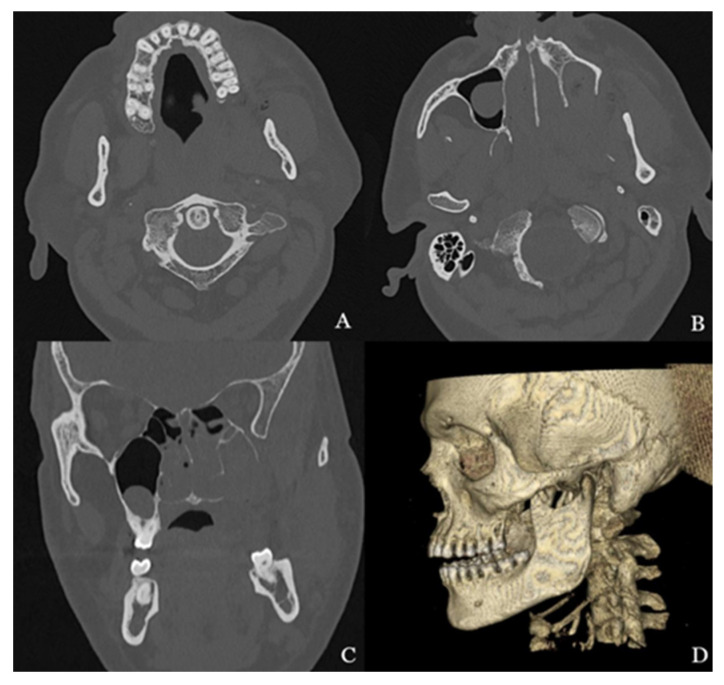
CT scans showing the bone defect in the maxilla after the extraction of tooth 28 ((A,B) axial sections, (C) coronal section), and a 3D reconstruction of the defect (D). The vascular abnormalities are not visible.
